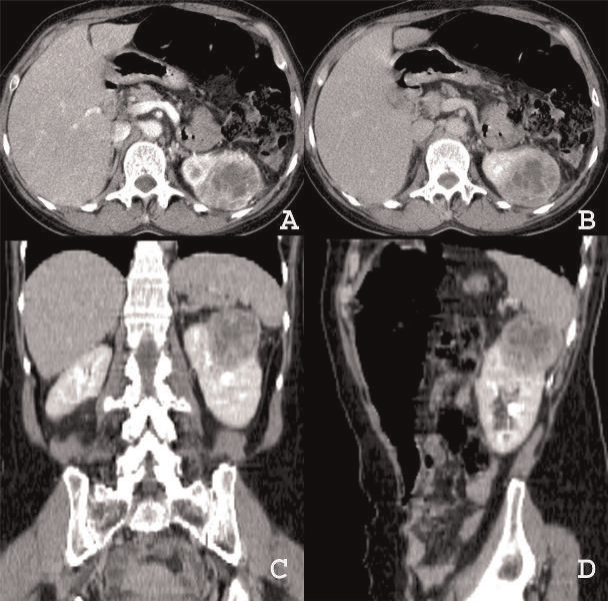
Abdominal CT scan demonstrating a left-sided mass in a 56-year-old woman. The mass was well-defined, exophytic-appearing to arise from the cortex—and heterogeneously and avidly enhancing. (A) Corticomedullary and (B) Nephrographic phases show typical hypervascularity of the tumor. (C) Coronal and (D) Sagittal multiplanar reformatation during excretory phase.
